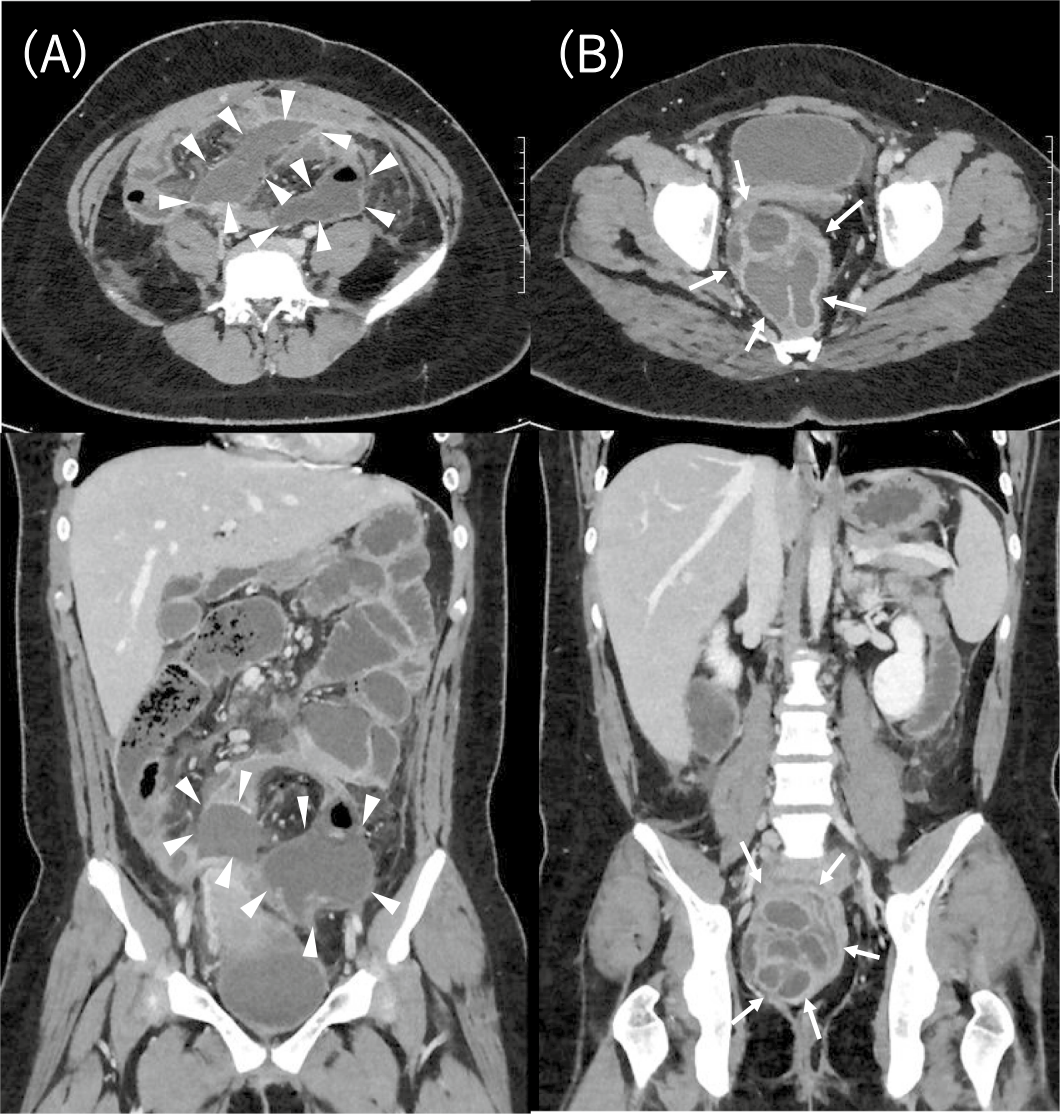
CT. A Abdominal abscess (white arrowhead). B Pyosalpinx (9 × 8 × 7 cm) (white arrow)
